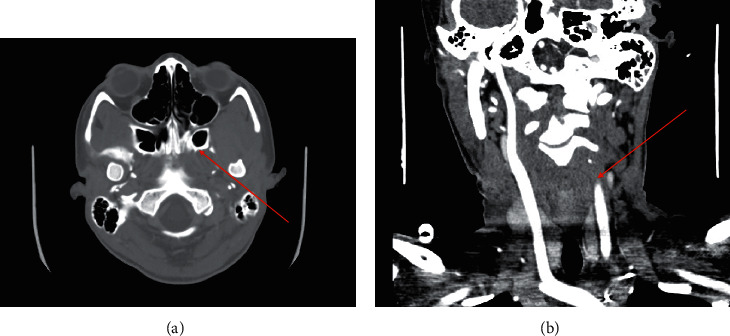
CT angiography. (a) CT angiography venous phase of the head, demonstrating left CST. (b) CT angiography head demonstrating left ICA occlusion.
